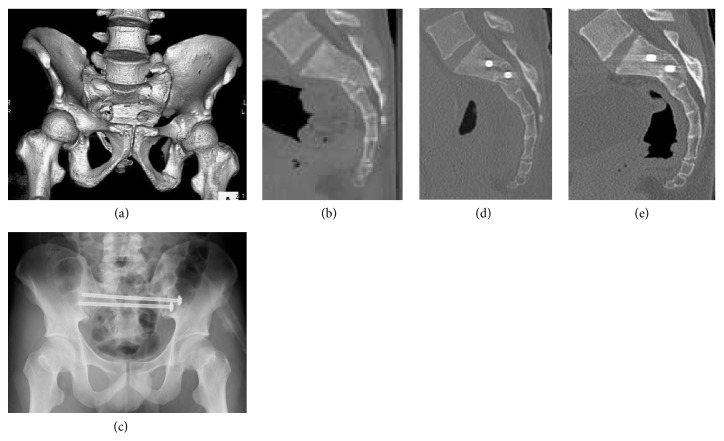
Case 1. A 25-year-old man who was injured from a fall from a height underwent S1 and S2 TSTI partial threaded screw fixation for a Roy-Camille type 2 U-shaped transverse sacral fracture. (a) Preoperative 3-dimensional CT reconstruction of the pelvis is shown. (b) Preoperative CT scan of the sacrum in the sagittal plane is shown (preoperative sacral kyphotic angle: 33°; fracture translation: 10 mm). (c) Postoperative anteroposterior radiograph of the pelvis is shown. (d) Postoperative CT scan of the sacrum in the sagittal plane is shown (postoperative sacral kyphotic angle: 17°; fracture translation: 2 mm). (e) Final follow-up CT scan of the sacrum in the sagittal plane is shown. Slight reduction loss was observed.
